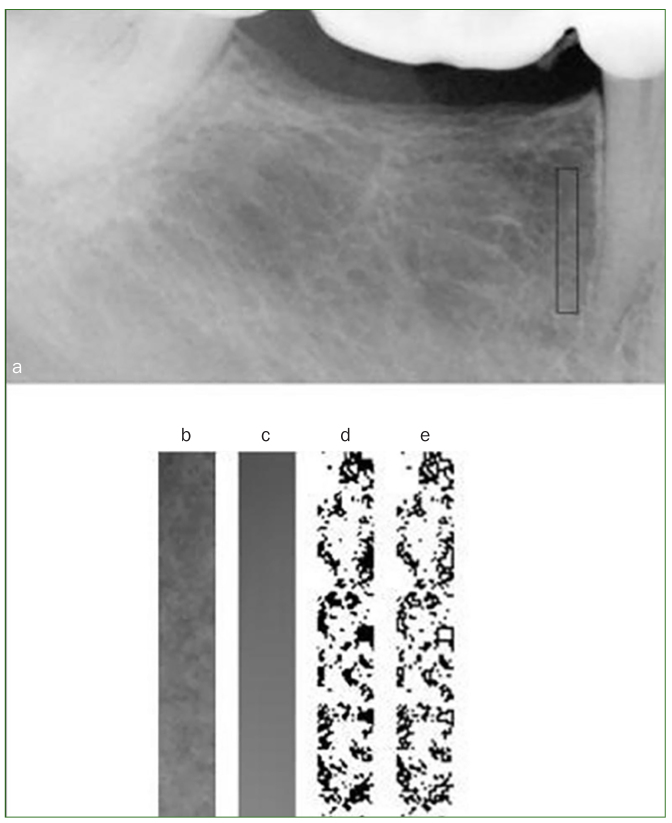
The original radiograph with a region of interest (ROI). (a) The selected ROI. (b) The result of blurring this region. (c) The result of subtracting B from A and adding 128. (d) Inverted binary version of the image. (e) Outlines of the trabeculae are obtained.
